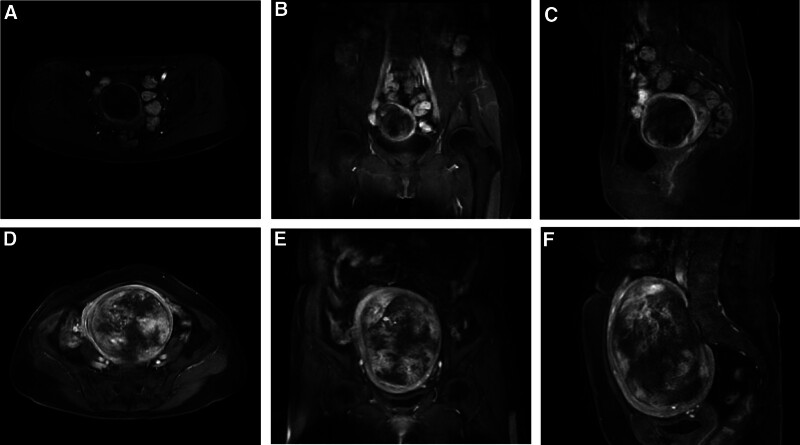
Magnetic resonance imaging (MRI) of uterine inflammatory myofibroblastic tumor (UIMT). A mass with a size of about 6.58 × 6.92 × 6.36 cm (A–C) and 12.2 × 9.8 × 14.7 cm (D–F) occupied in the wall of uterus. Transverse (A and D), coronal (B and E), and sagittal (C and F) sections are observed in the images.
